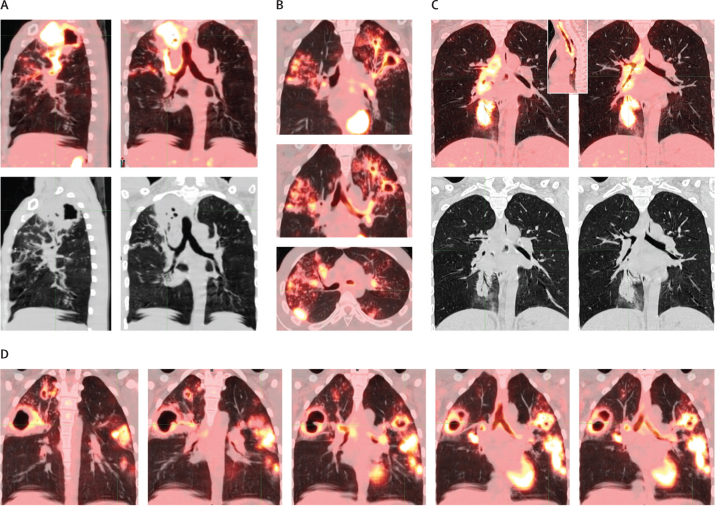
Examples of bronchial inflammation on baseline PET-CT scan (A) Inflammation from right S2 segment cavity flows down bronchus, which is better seen on CT alone (black and white image), into the right main bronchus and trachea. (B) Inflammation from left upper lobe cavities flows down into the left main bronchus. Although inflammation is not visualised crossing the carina, there is a direct bronchial connection to right S2 and S3 segment lesions. (C) Right lower lobe S7 segment consolidation with inflammation travelling up the right main bronchus and trachea to the pharynx (inset image shows the the trachea to pharynx; black and white image is CT alone). (D) Right and left S3 segment cavities with bronchial inflammation connecting the two across the carina, spreading inferiorly to the left S6 segment. Figures A–C are from the PredictTB study. Figure D is from a patient in a different study (NexGen EBA: Radiologic and Immunologic Biomarkers of Sterilizing Drug Activity in Tuberculosis, NCT02371681).
